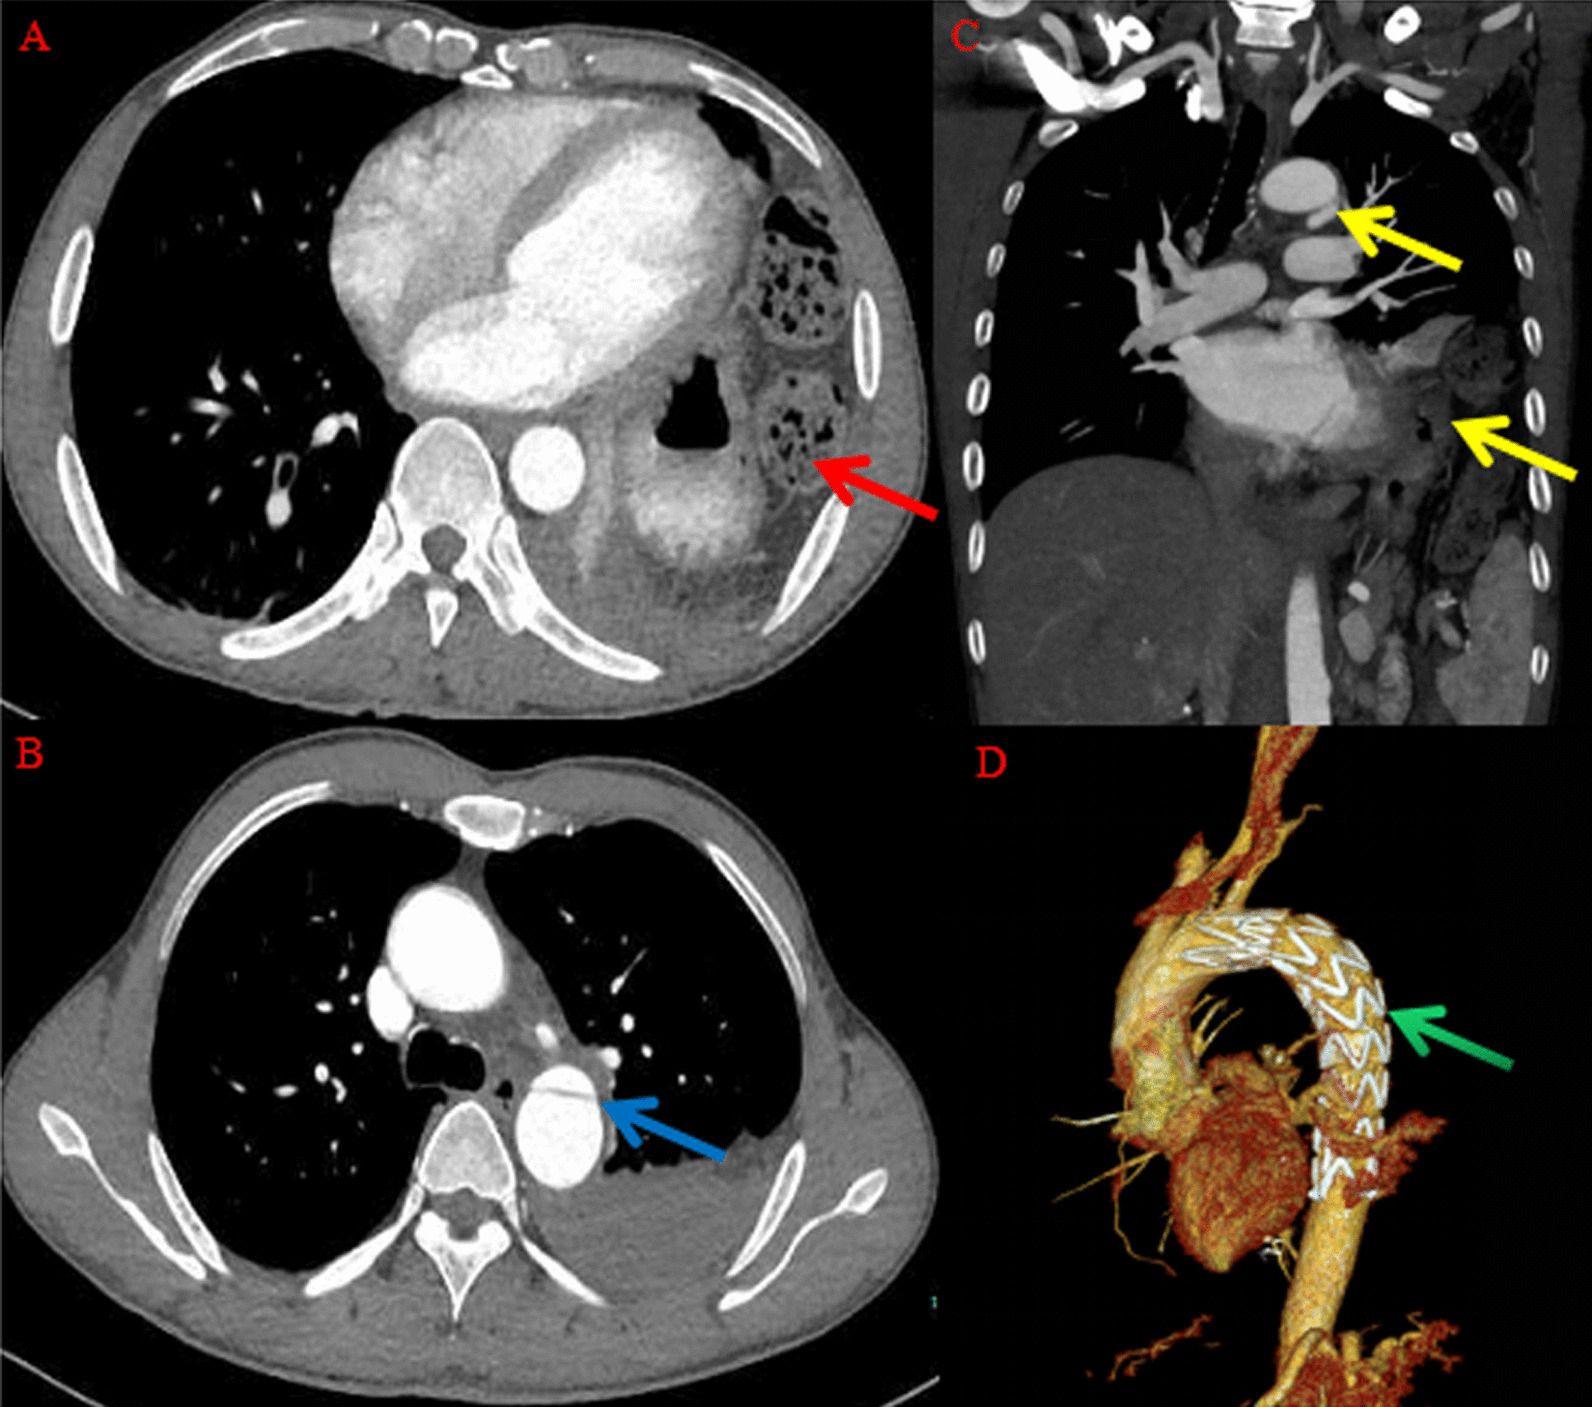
Vascular CTA imaging scan of Case 3. A Vascular CTA suggested herniation of the left abdominal contents into the thoracic cavity with consolidation of the left lower lobe (red arrow). B Intimomedial flap at the beginning of the descending aorta (blue arrow), and dissection was considered. C Vascular CTA showed traumatic diaphragmatic hernia with aortic dissection (Coronal, yellow arrow) D Femoral artery stent implantation with a thin mesh was performed (green arrow)
